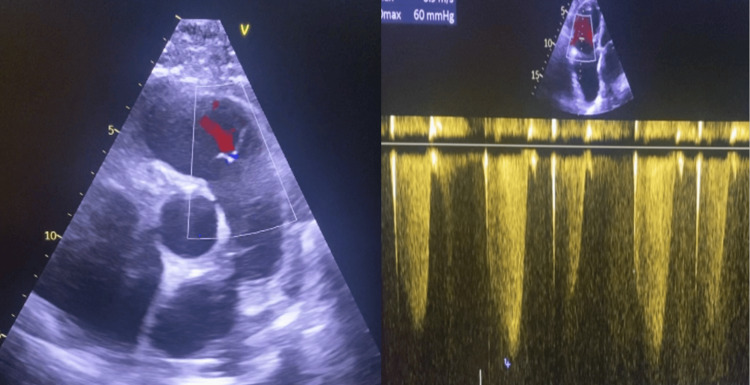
ETT in two-dimensional mode showing an apical section of four cavities centered on the right cavity It reveals the absence of the left pulmonary artery and notable dilation of the right atrium and right ventricle. The inferior vena cava velocity is 3.9 meters per second, and the right ventricle and right atrium gradient are 60 millimeters of mercury. ETT: echocardiogram
